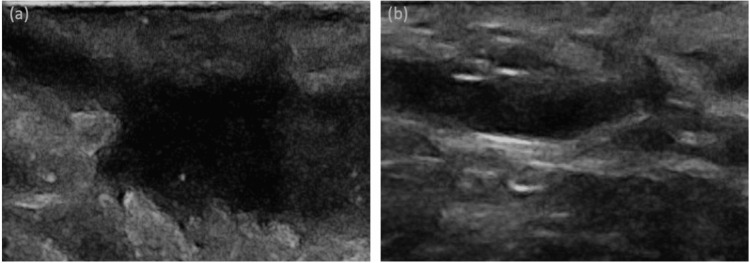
(a) and (b) Anechoic fluid collections and sinus tracts observed in the ultrasound scan of the lesions.
